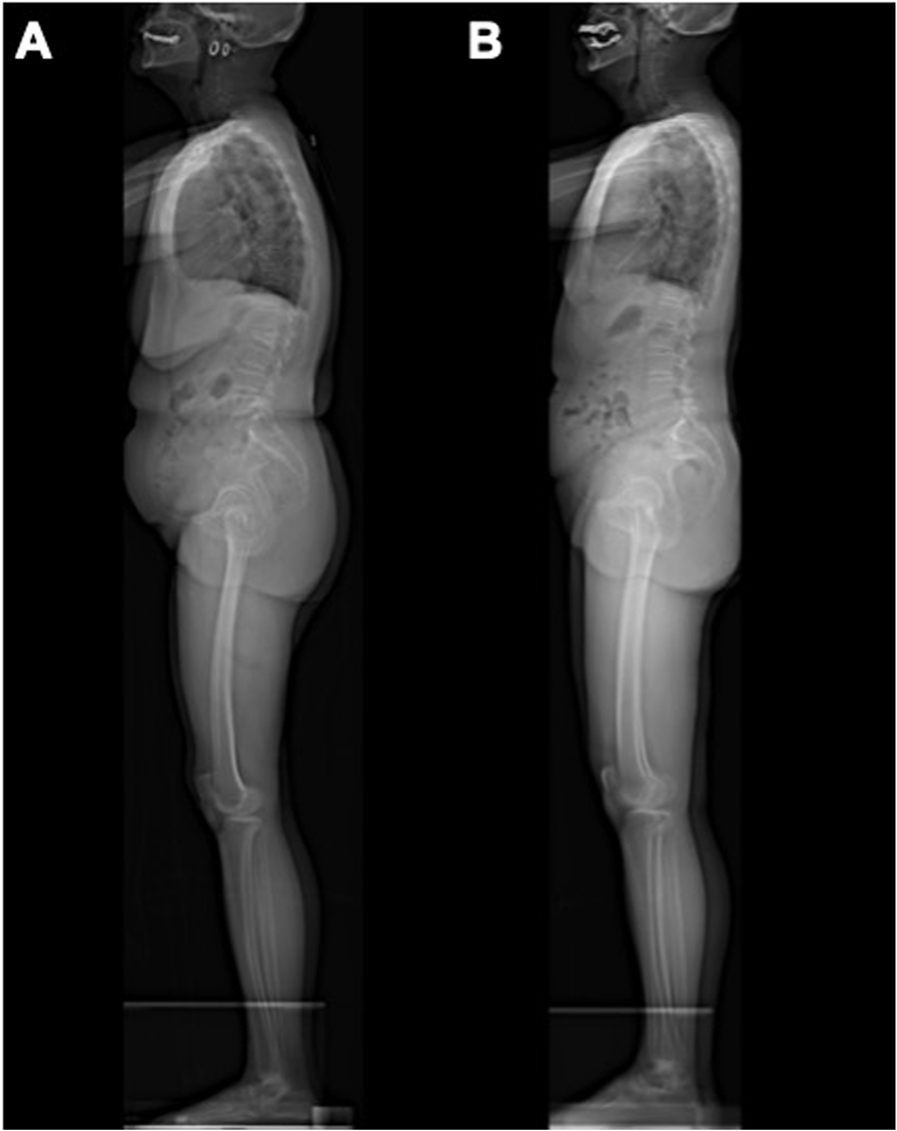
Representative radiographs of elderly adults recruited in this study. a 66 year-old female patient with VCF and b 66 year-old female patient without VCF in lateral views
